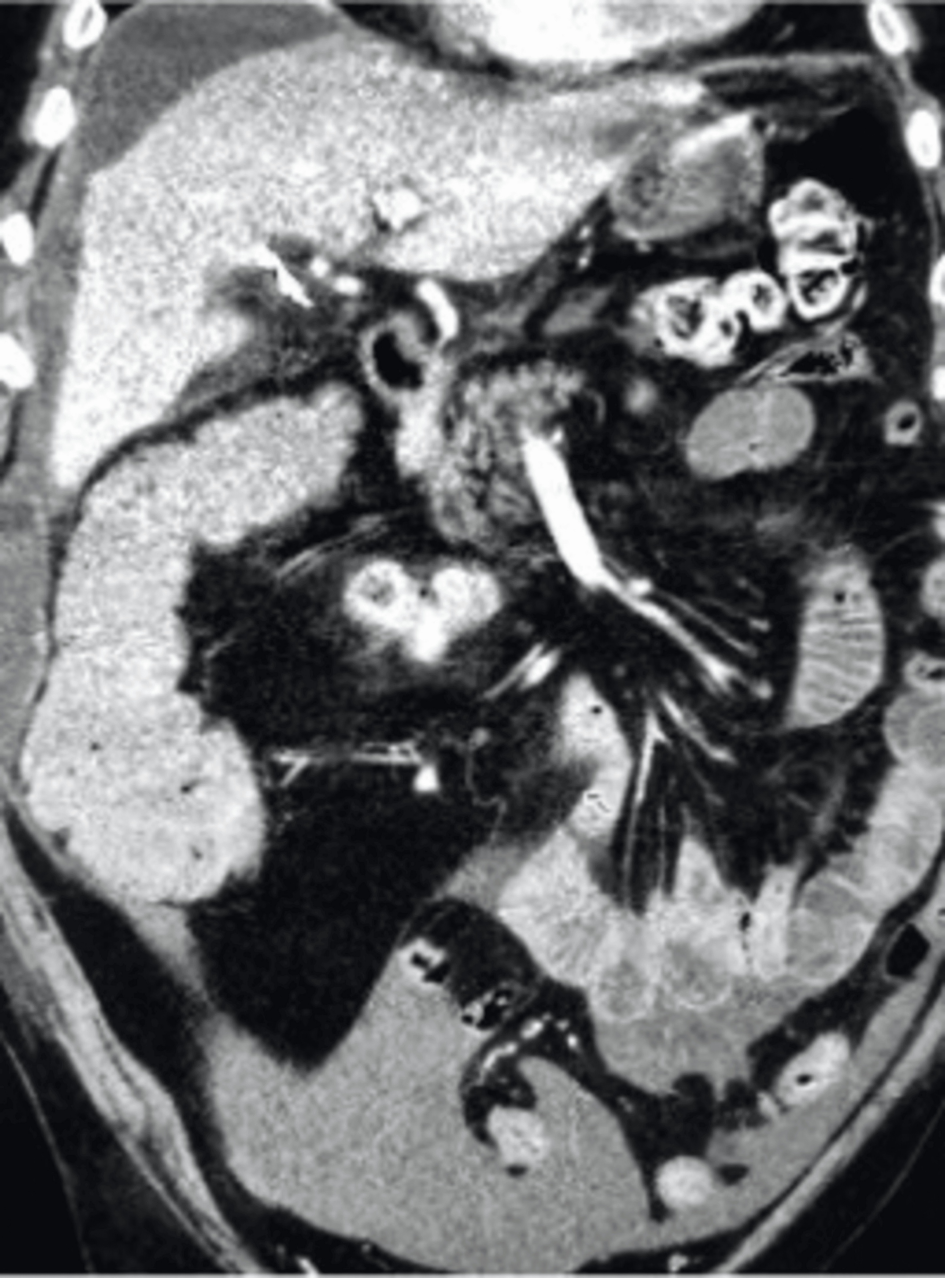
Fluid surrounding the liver and confirmation of surgical clip present at the site of cholecystectomy on CT imaging
